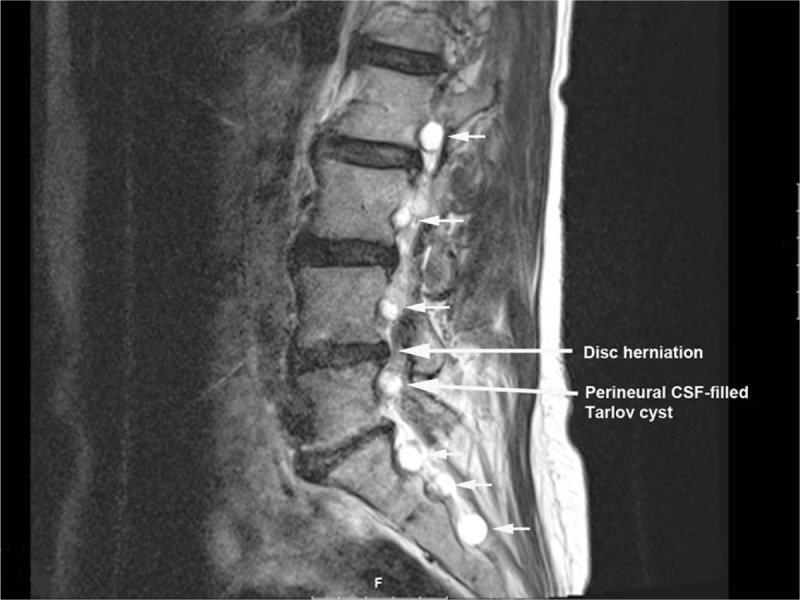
Multilevel foraminal Tarlov cysts (small arrows), in close proximity to disc herniation (large arrow) and Tarlov cysts in close proximity to approximate needle trajectory for transforaminal epidural injection technique (large arrow) on sagittal T2-weighted magnetic resonance imaging.
